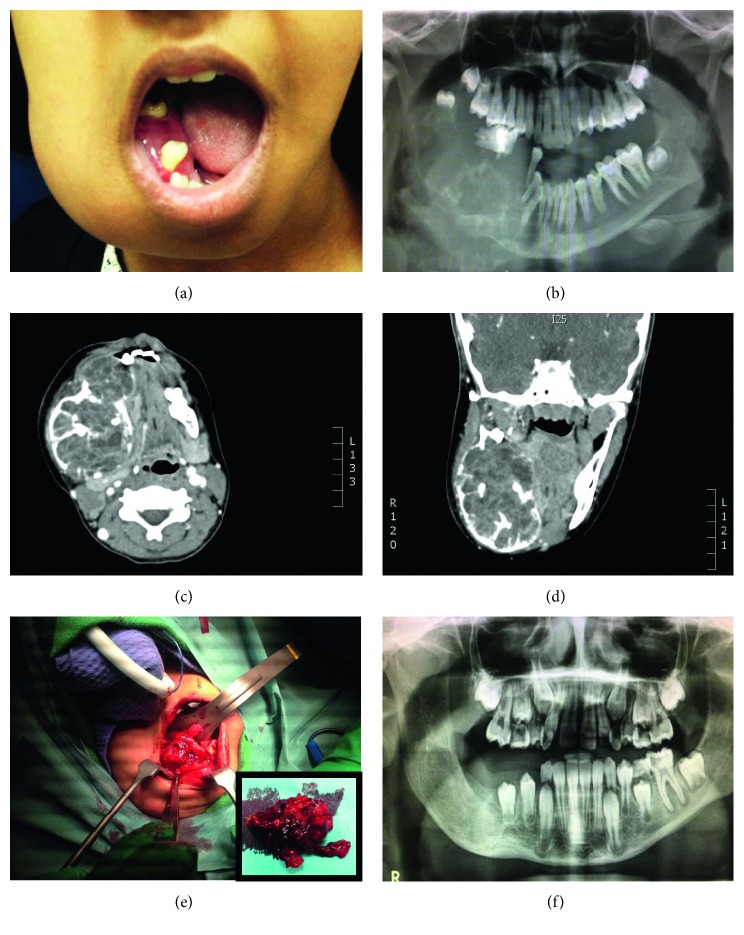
(a) A 12-year-old girl with right mandibular and neck mass displacing teeth all the way. (b) Panorex view that shows massive destruction of half of her mandible with extension into condyle, neck, and medial of right of mandible. (c) Axial and (d) coronal CT scan of neck and soft tissue that shows multiple septations along with wall enhancement within the lesion. (e) Large soft tissue tumor was visible intraoperatively. Gross examination of large hemorrhagic soft tissue mass measures 6 × 6 × 3 cm (inset). (f) Postoperative panorex view of the patient which shows complete healing of her bony defect with significant resolution in her unique facial deformity that resembles sarcoma.
